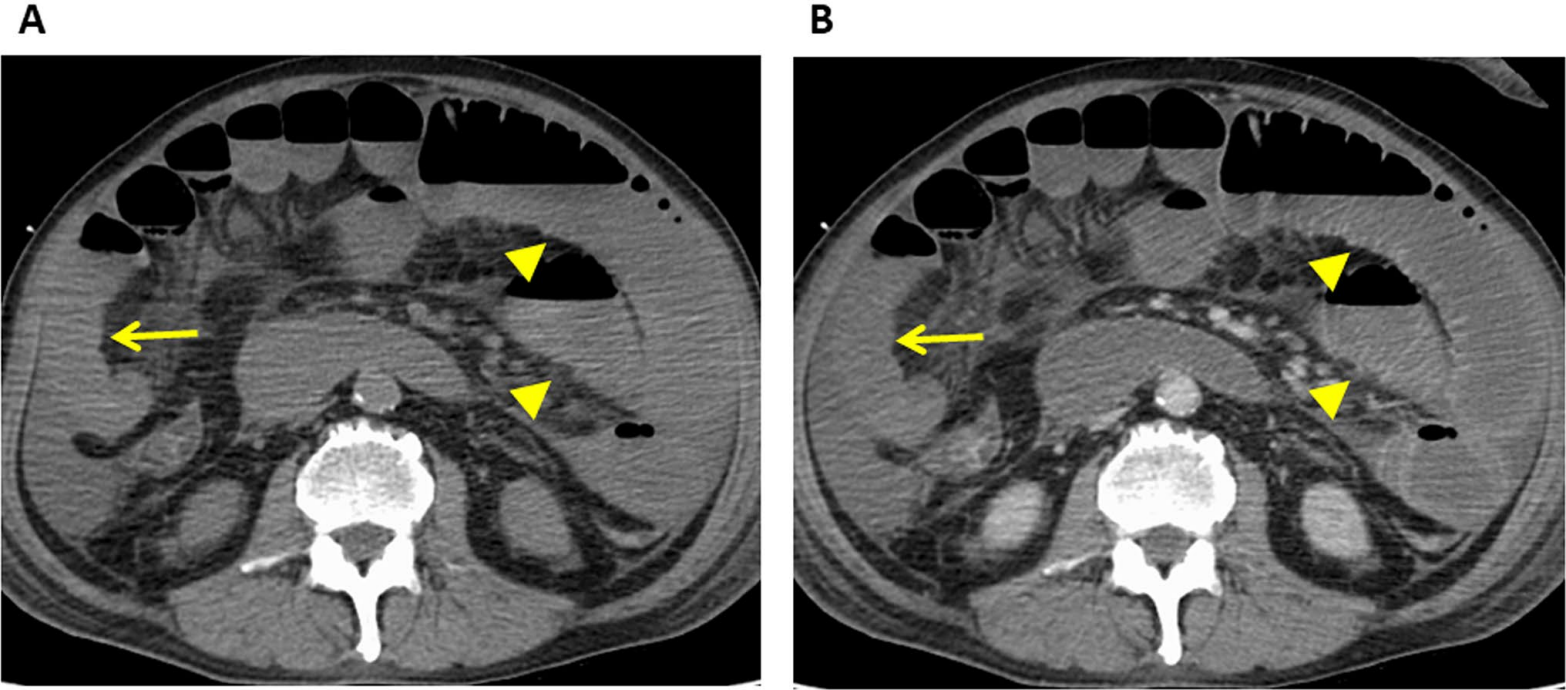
Non-occlusive mesenteric ischemia in a 50-year-old man (same patient as in Fig. 2). A Unenhanced CT image, B Contrast-enhanced CT image. The CT images show that the involved small bowel segments (arrows) are not contrast enhanced while the uninvolved segments are contrast enhanced (arrow heads)
